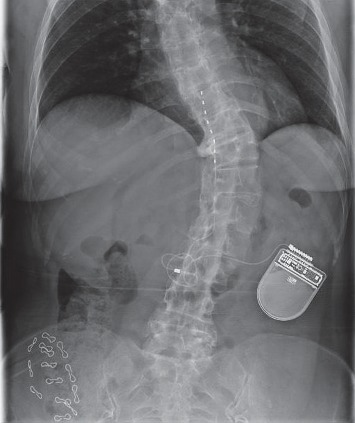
X-ray image of the thoracic and lumbar spine. The degree of scoliosis and the presence of the linear electrode of the spinal neurostimulator at low thoracic level can be seen.
